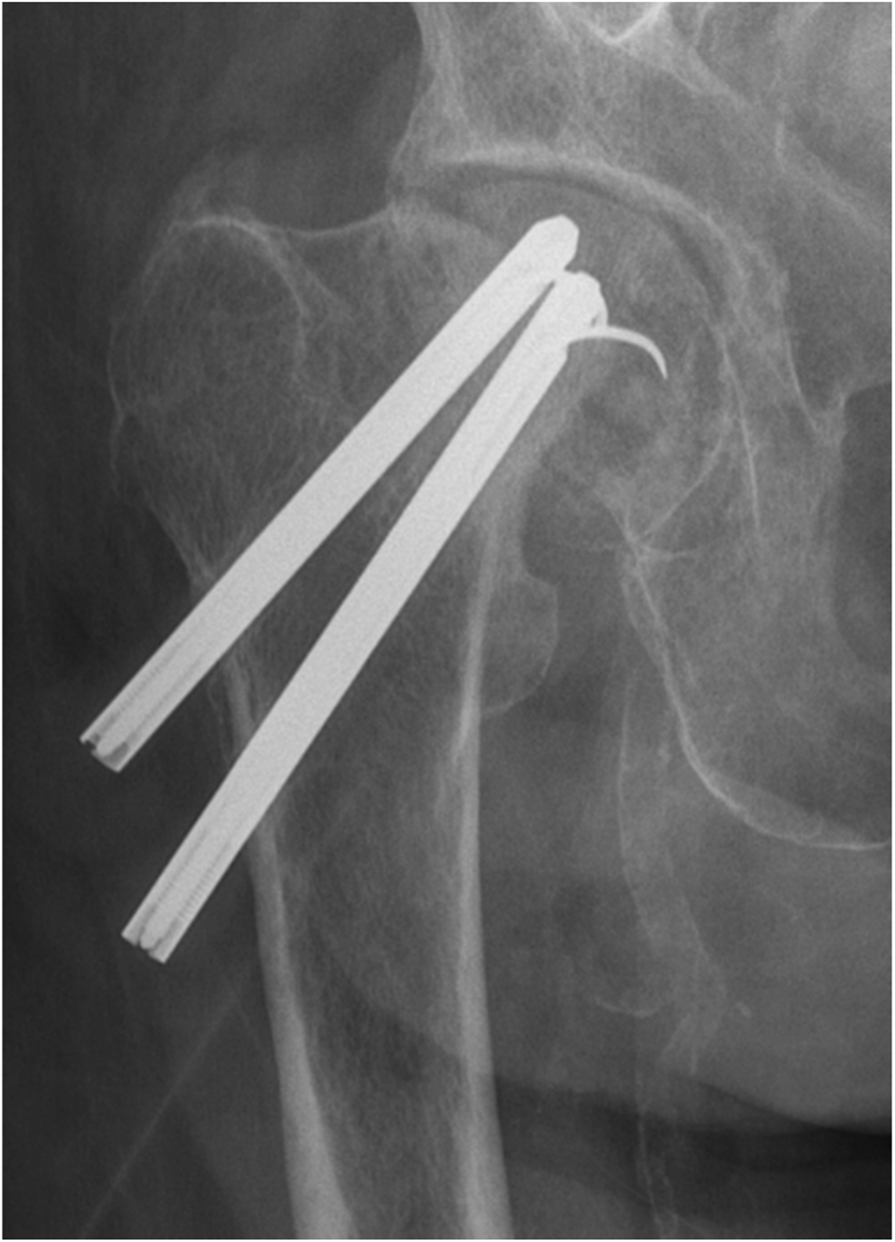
Plain radiographs of an occult femoral neck fracture treated with internal fixation and who later developed a treatment failure
